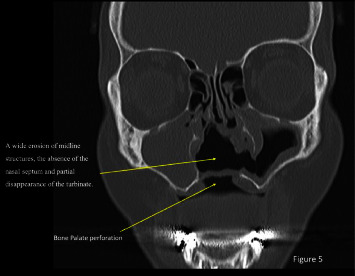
Coronal section of the CT scan revealed a wide erosion of midline structures, the absence of the nasal septum, and partial disappearance of the turbinates. Note the palatal perforation that establishes an oronasal communication.
